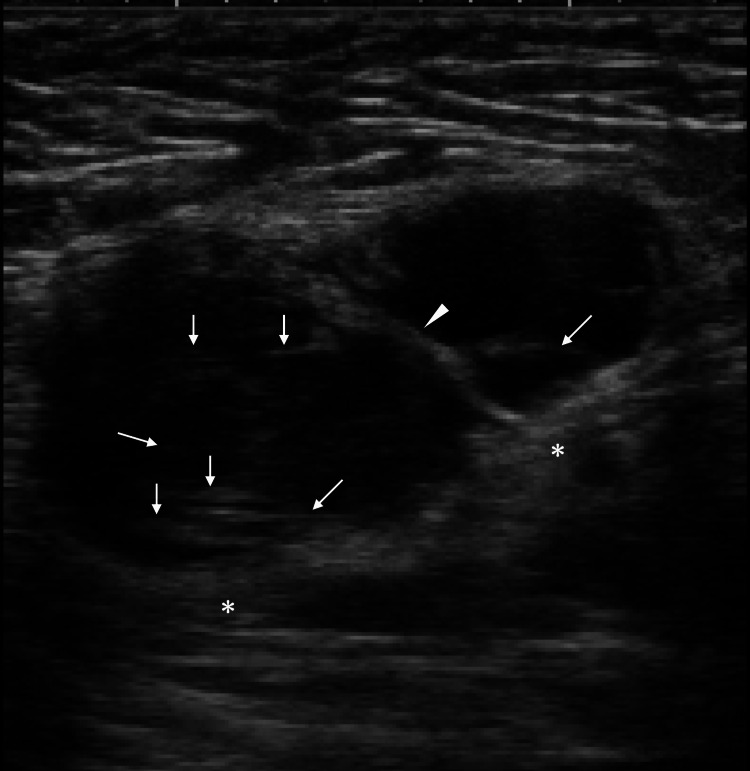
Ultrasound findings Ultrasound showed a lobulated mass deep in the axilla with 21×19×15 mm in size, distinct margins, slight peri-tumoral vascularity, very low internal echoes, enhanced posterior echoes (asterisk), an intra-tumoral septum (arrowhead), and scant punctate and linear high echoes (arrows)
